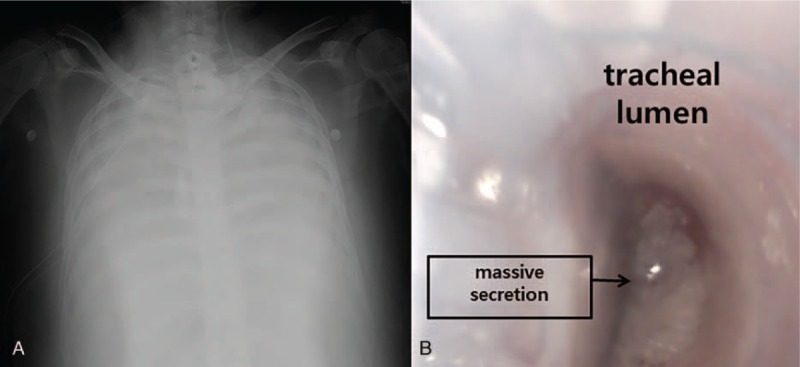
Perioperative images of the patient. A, Preoperative chest x-ray, showing severe diffuse lung consodiation. B, Bronchoscopic finding, showing massive pulmonary secretion within the conventional double-lumen endobronchial tube.
